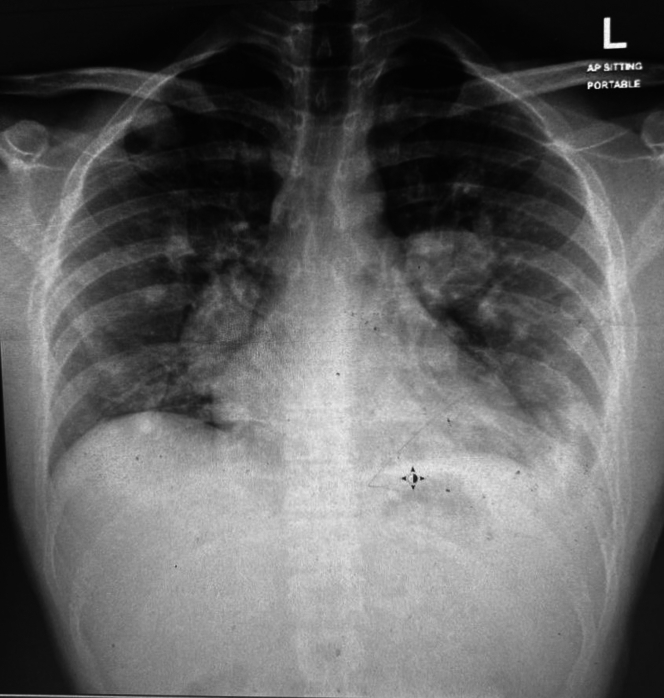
Chest Radiograph Showing Multiple Bilateral Lung Nodules
